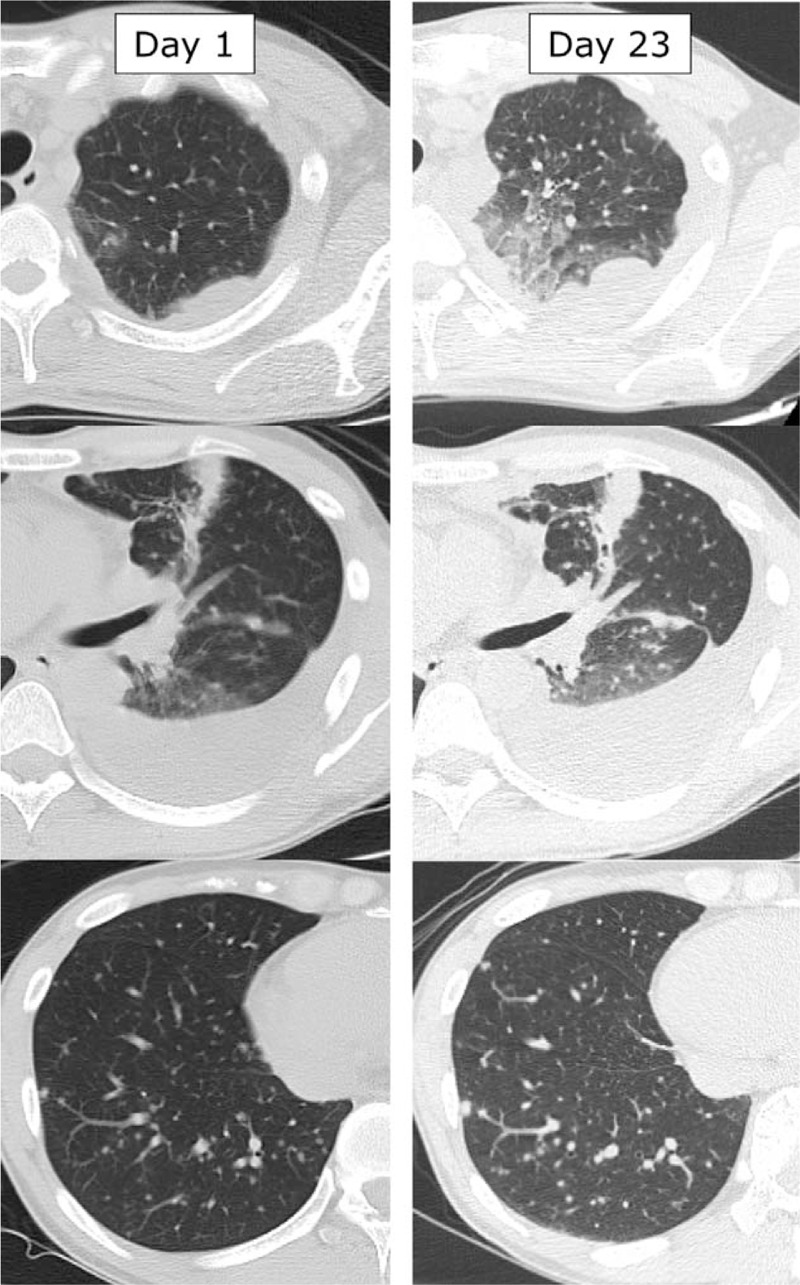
Computed tomography scans of the patient's chest. Day 1: CT scan on the day of starting antituberculous therapy. Day 23: CT scan 23 days after starting antituberculous therapy. The images show newly formed ground-glass opacities, increased pleural effusion on the left side, and enlarged miliary pulmonary nodules.
